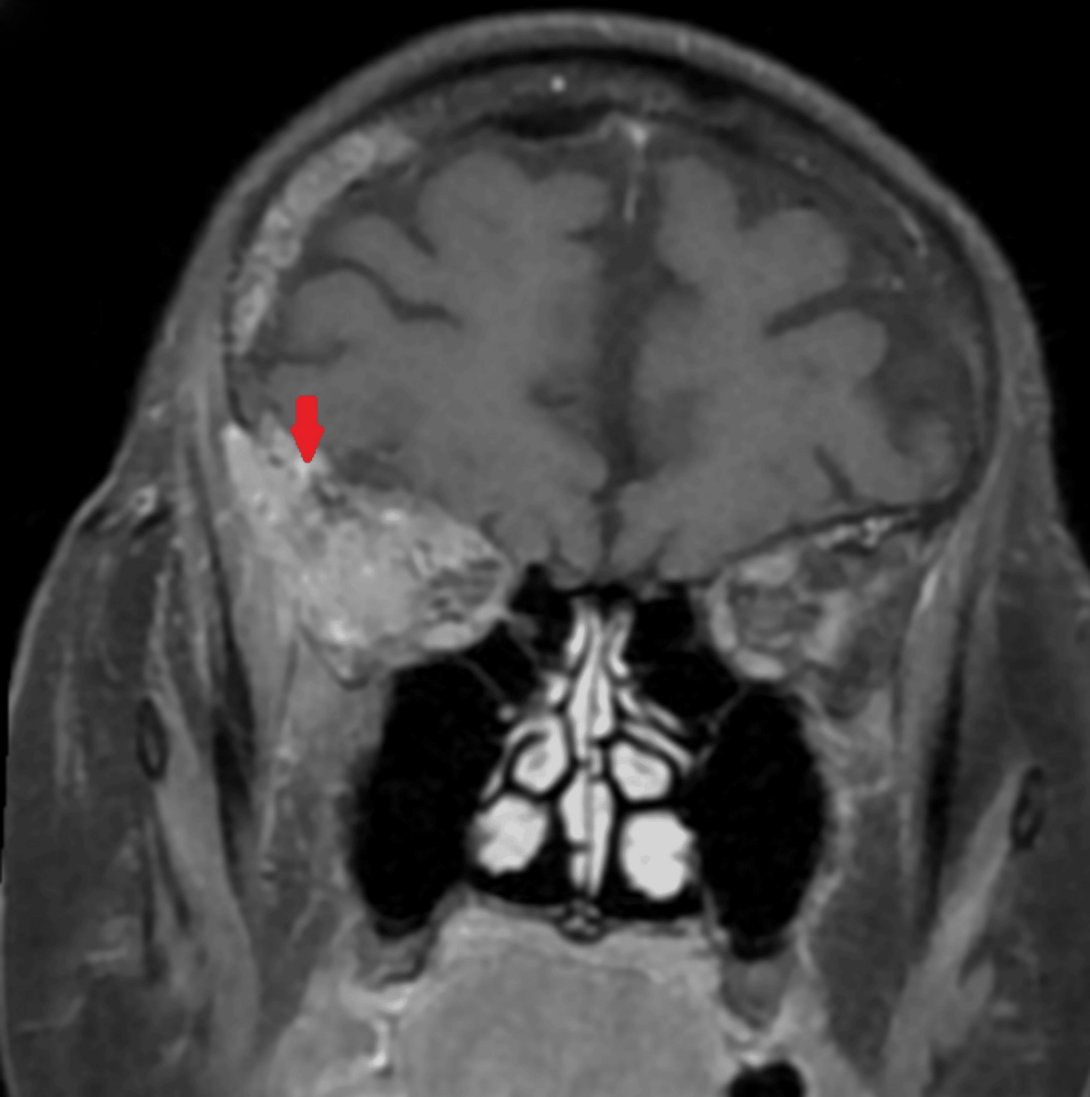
MRI orbit and brain (coronal view) on the first presentation MRI: magnetic resonance imaging
